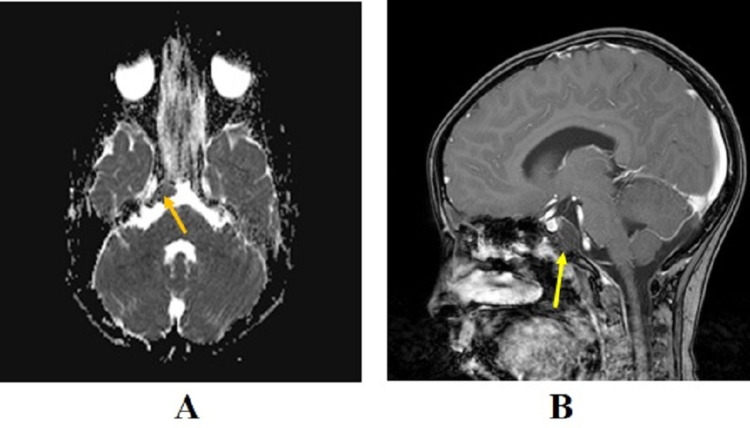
Postoperative 12-month follow-up MRI scans of the patient Postoperative 12-month follow-up MRI scans of the patient with (A) diffusion-weighted image (DWI) showing recurrent lesion with restricted diffusion on the retroclival prepontine cistern (orange arrow); and (B) sagittal post-gadolinium T1 weighted image (T1WI) showing no tumor enhancement (yellow arrow).
